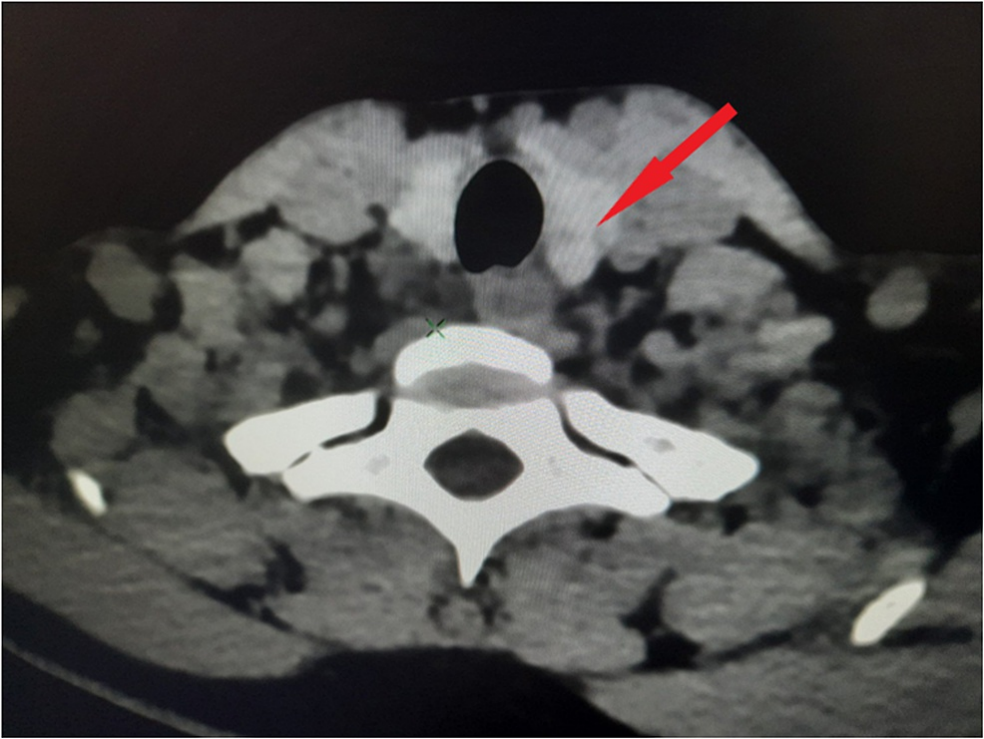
Four-dimensional computed tomography scan showing parathyroid adenoma (red arrow).
